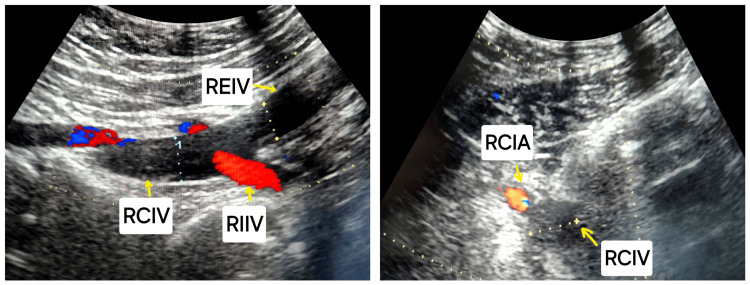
Doppler ultrasound revealing the absence of blood flow from RCIV and REIV RCIV: right common iliac vein; REIV: right external iliac vein; RIIV: right internal iliac vein; RCIA: right common iliac artery
